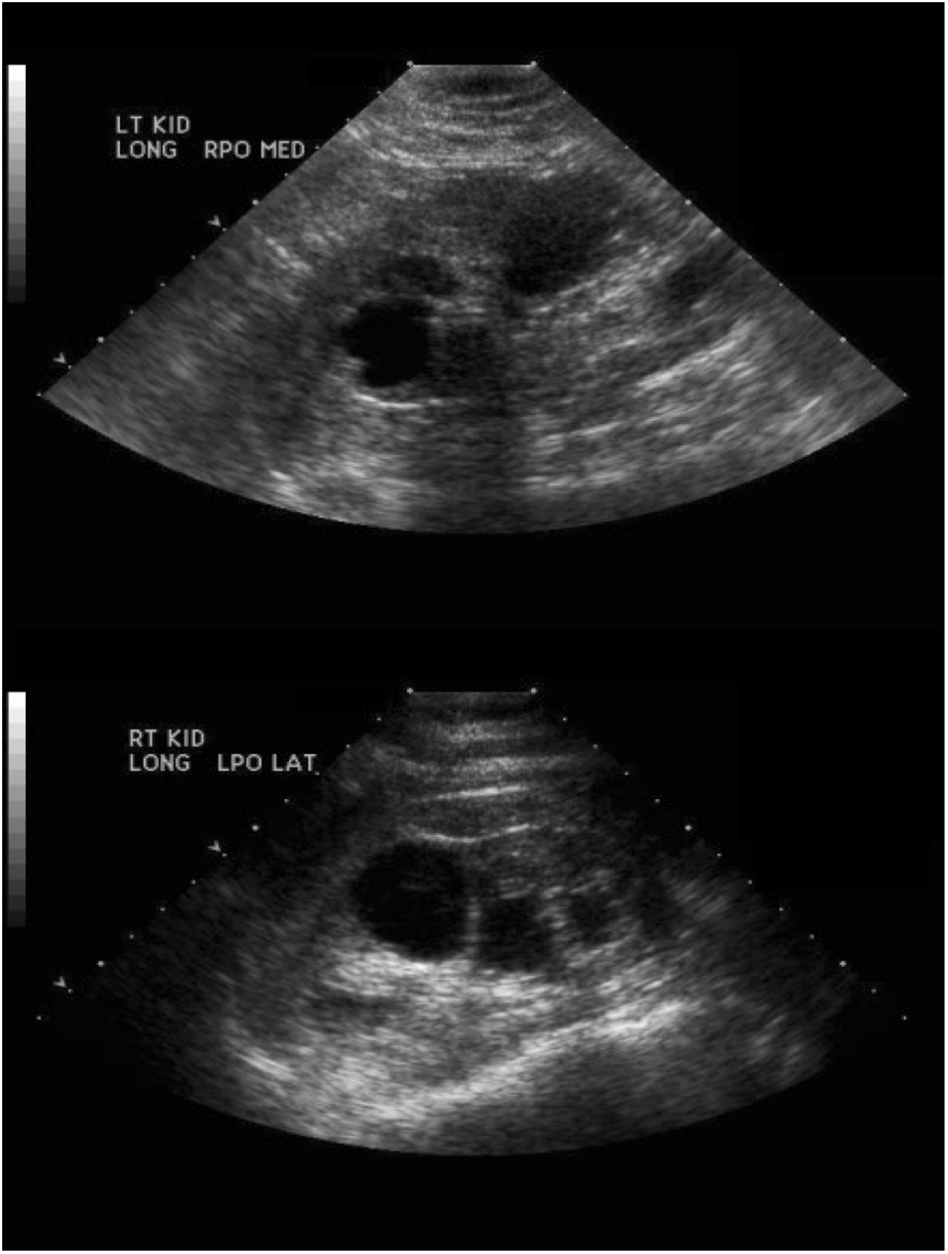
Renal ultrasound showing multiple bilateral cysts
